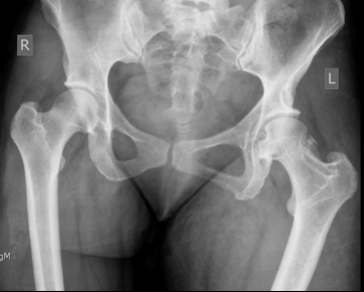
Radiograph of pelvis demonstrate left hip to be in joint.
